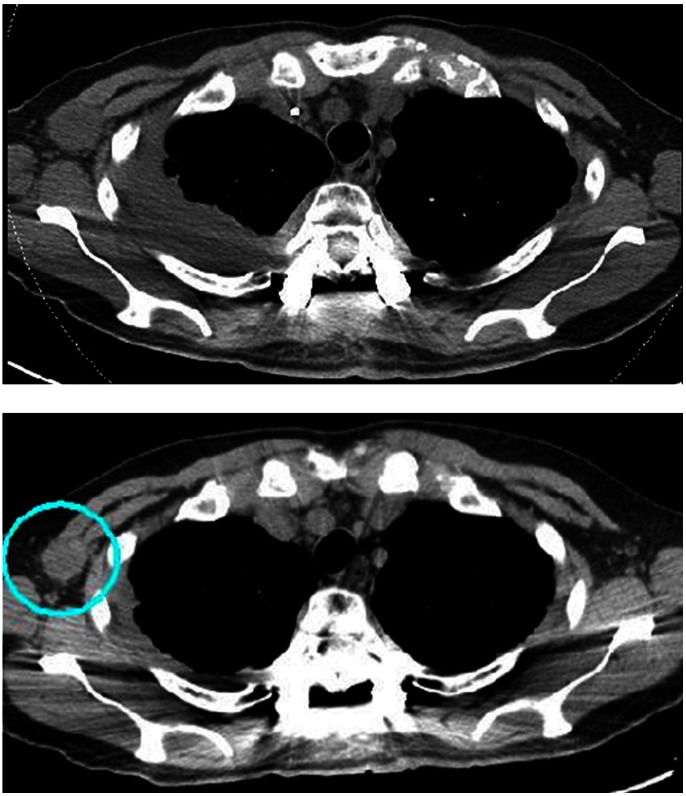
Postoperative computed tomography of the lung (Top; immediately after TES, Bottom; 1 month after TES). Right axillary lymph node metastasis is reduced. A circle shows reduced lymph node of right axilla.
